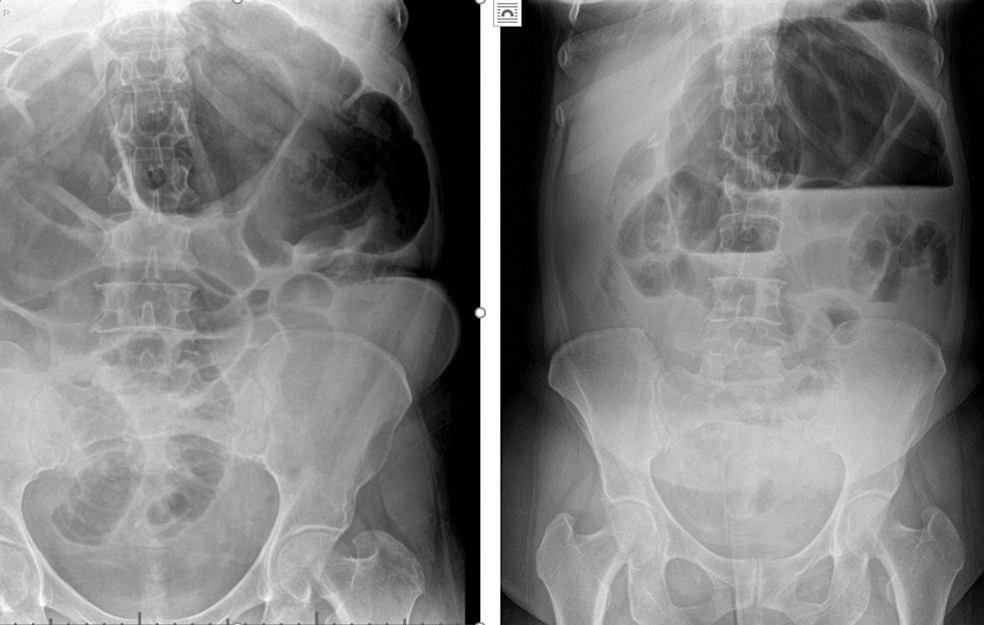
Plain X-RAY of the erect abdomen (on the left ) showing distended loops of the large bowel extending across the upper abdomen, and supine (on the right) showing fluid levels.
